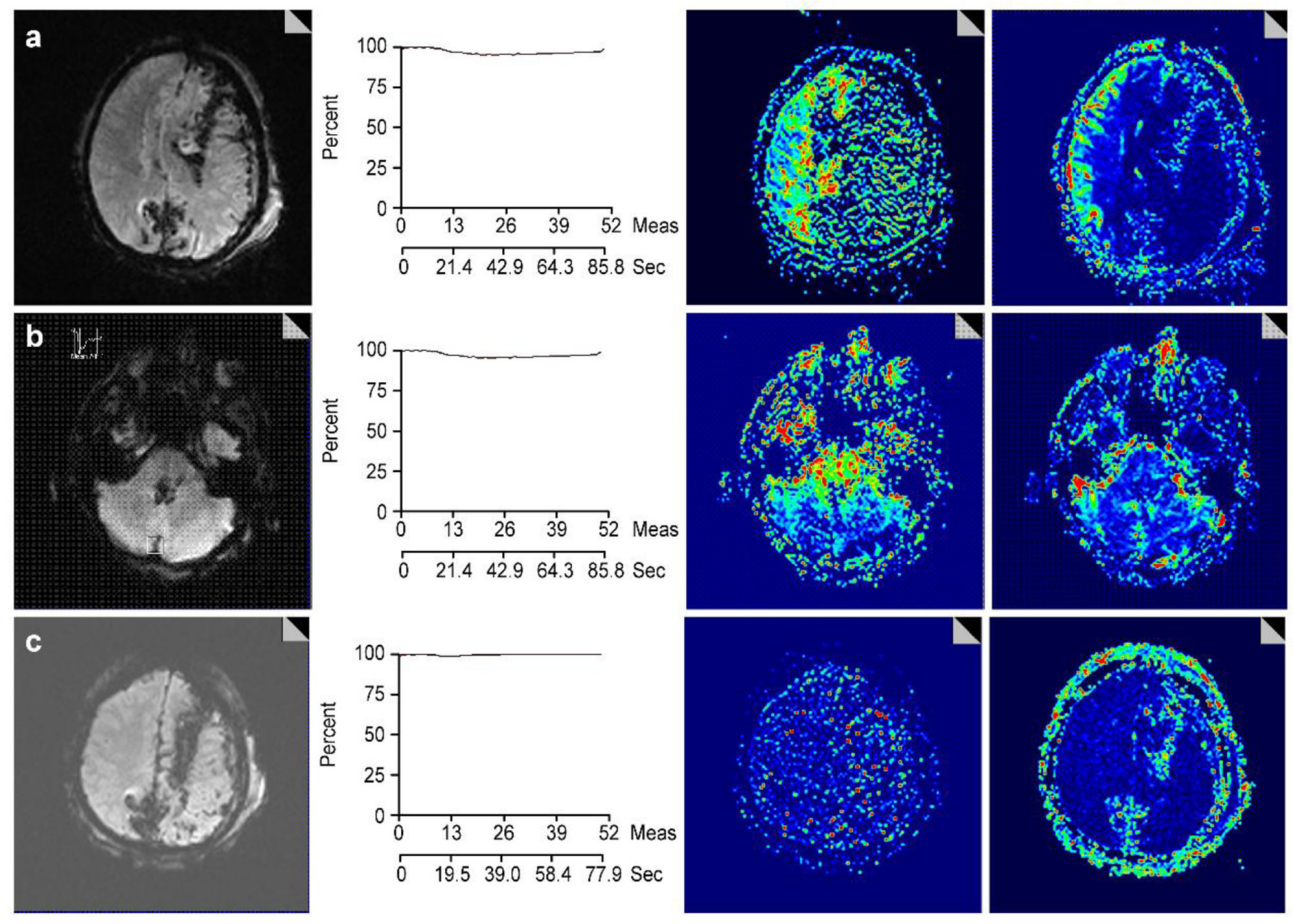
A 28-year-old man was admitted to the intensive care unit after a firearm wound to his left cranium. His Glasgow coma score was 3. a) Axial images magnetic resonance images at the level of the centrum semiovale. From left to right panels: The gradient echo MR image shows the hypointense tractus of the bullet in the left cerebral parenchyma and a midline shift to the right. The perfusion signal-time course shows the presence of diminished perfusion. The mean transit time (MTT) and cerebral blood flow (CBF) maps show perfusion in the right cerebral hemisphere and absence of perfusion in the left hemisphere. b) Axial images were acquired at the level of the pons in the same order as in a): Both the MTT and CBF maps show the presence of perfusion in the cerebellum and the pons. c) A second cerebral MR perfusion examination was performed one day later. Axial images at the level of the centrum semiovale in the same order as in a): The tractus of the bullet in the left cerebral parenchyma remain visible, but the midline shift is less pronounced. The perfusion signal-time course graphic has flattened and both MTT and CBF maps show absence of perfusion in both cerebral hemispheres.
